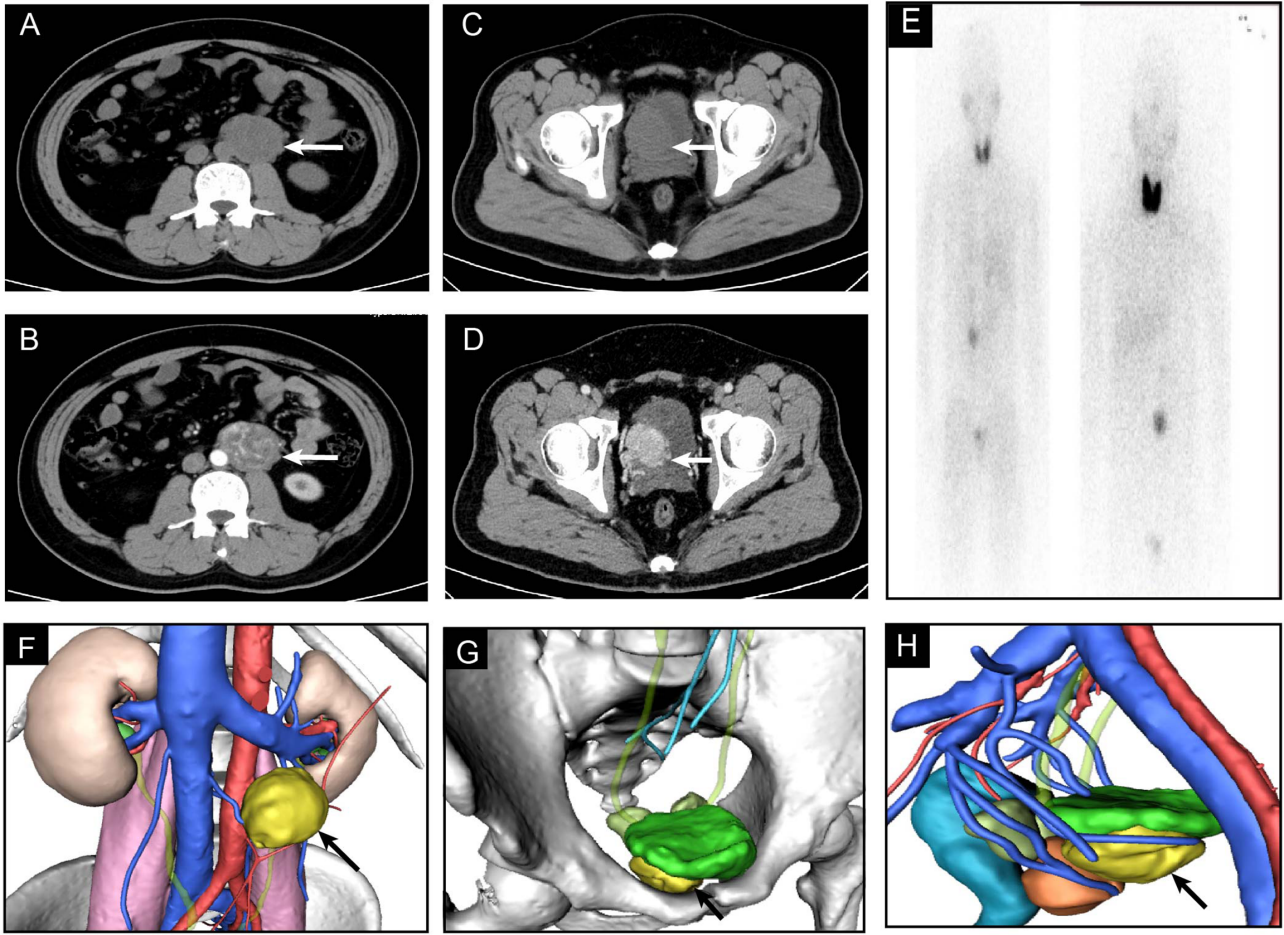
The enhanced CT and 3D model of PGLs. (A, B) Plain and enhanced CT images of PGL in abdomen. (C, D) Plain and enhanced CT images of PGL in pelvis. (E) MIBG scintigraphy. (F–H) 3D model of CT imaging.
